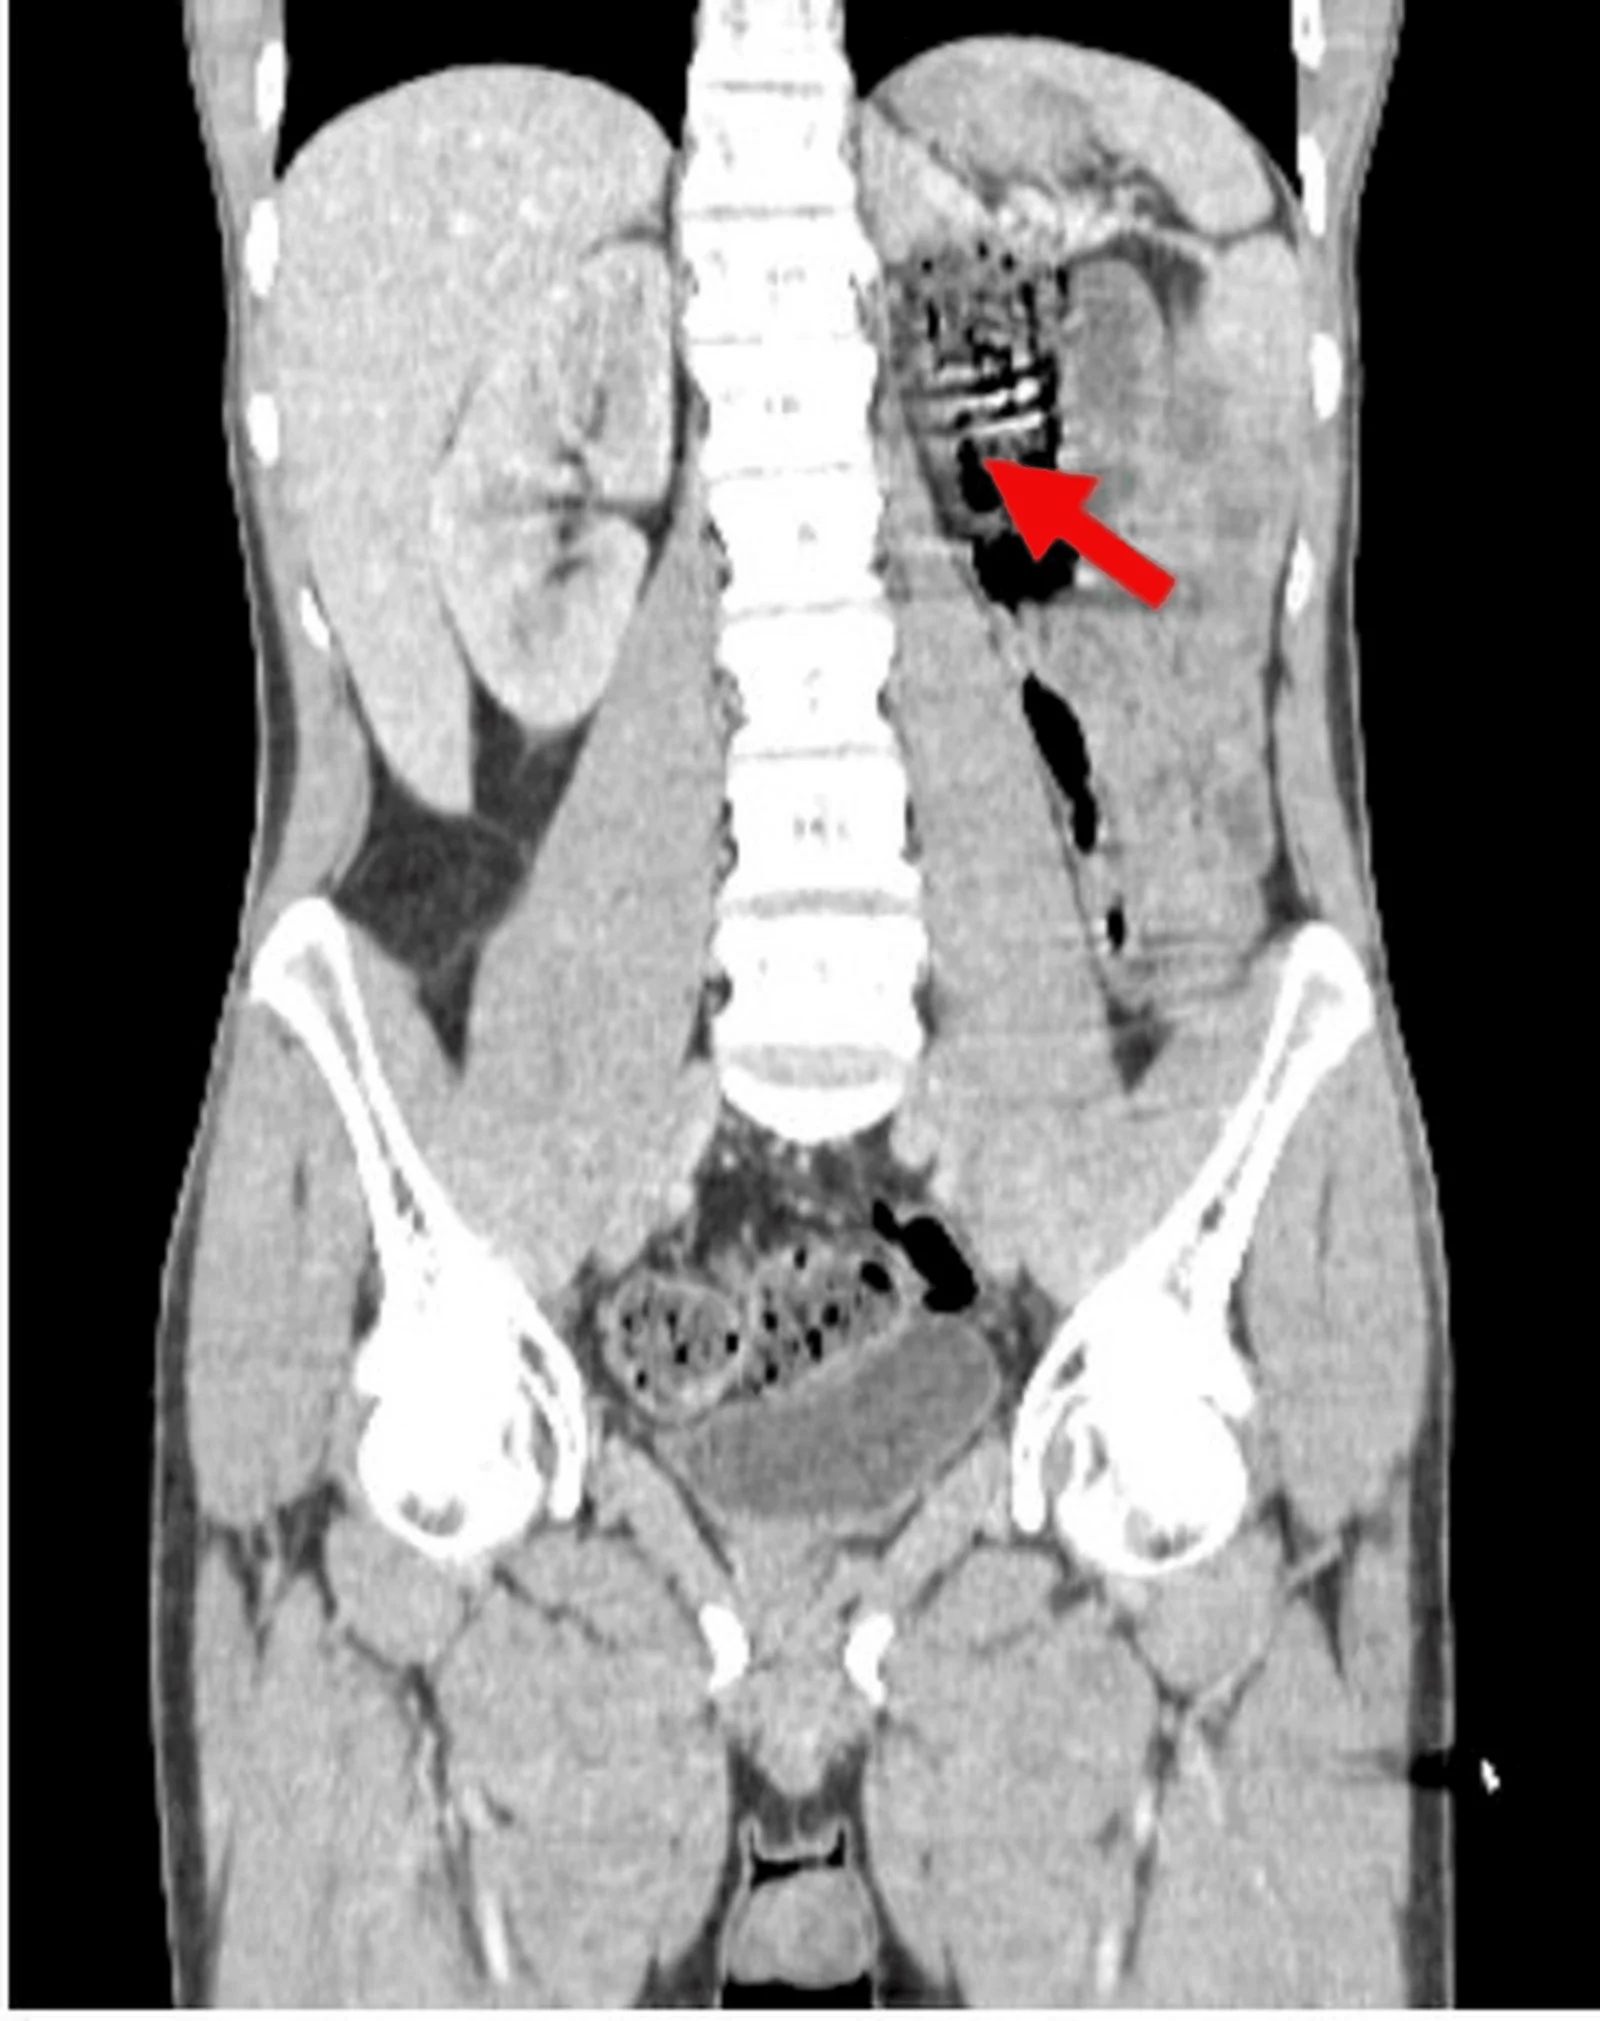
Coronal contrast-enhanced computed tomography (CT) image of the abdomen and pelvis demonstrating complete absence of the left kidney in the renal fossa (red arrow), with no evidence of ectopic renal tissue, consistent with ipsilateral renal agenesis.
